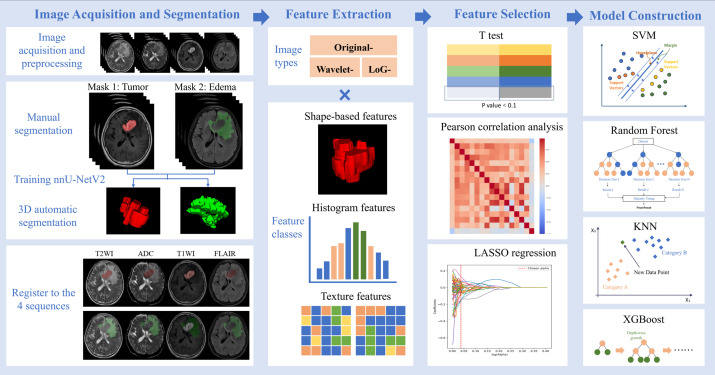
Study design: MRI acquisition and preprocessing, automated segmentation, radiomics feature extraction and selection, and model construction.
